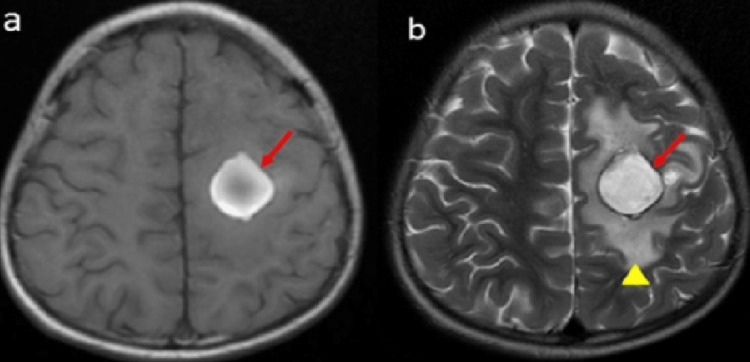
MRI showing T1 (a) and T2 (b) hyperintense well defined rounded lesion with surrounding edema in left centrum semiovale corresponding to left MCA territory suggestive of late sub-acute hemorrhage. MCA - middle cerebral artery
